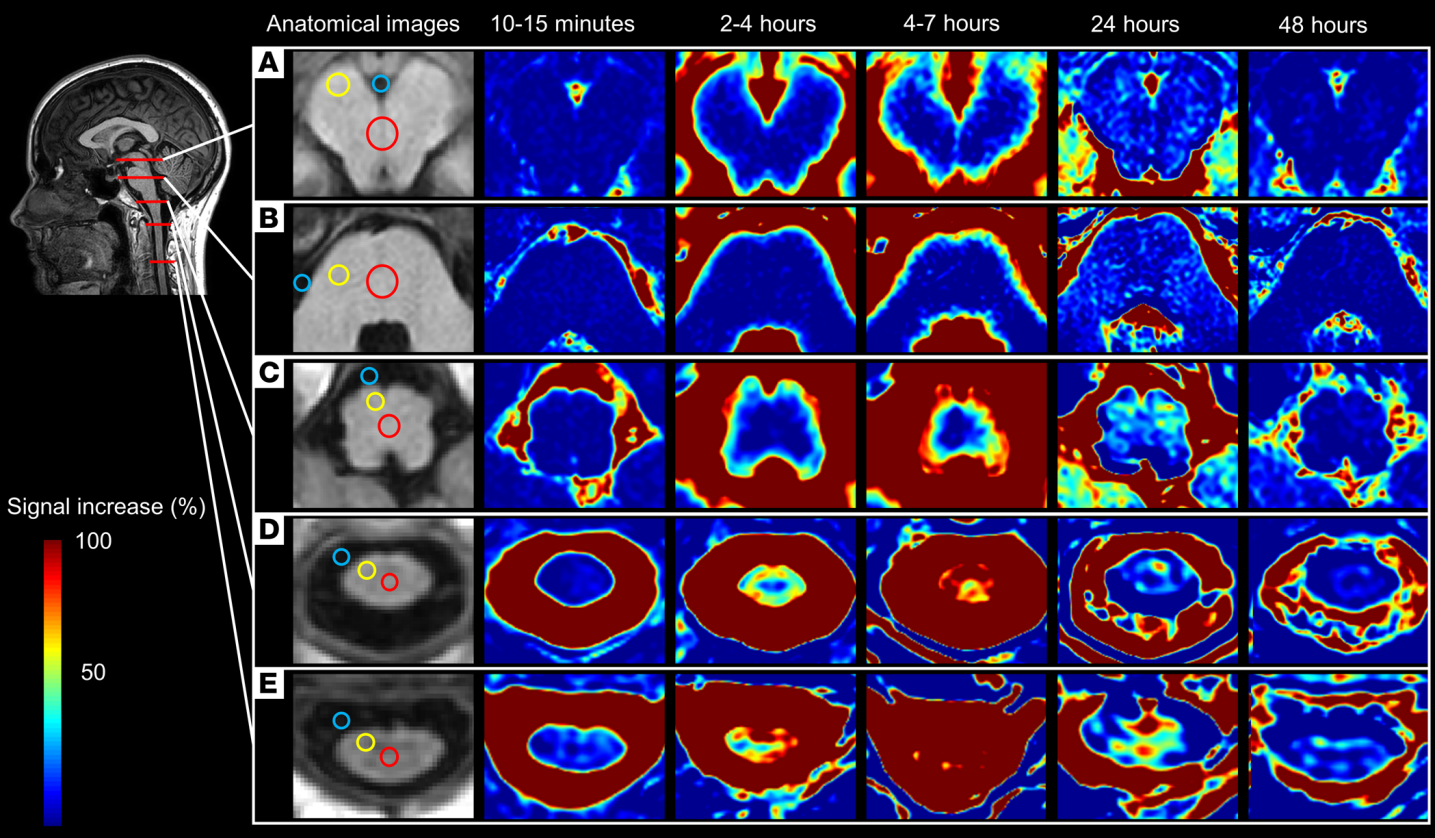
Tracer distribution over time and regions of interest for one reference patient. (A–E) Images of all time points and anatomical regions: mesencephalon (A), pons (B), medulla oblongata (C), C1 level of the spinal cord (D), and C3-level of the spinal cord (E). The 3D T1 images for all time points were first registered using Philips IntelliSpace, version 12.1. The signal in the T1-weighted images before contrast was then subtracted from the normalized signal for each time point. The tracer moves in a centripetal direction with higher and earlier detected levels of tracer in the superficial regions compared with the deep regions. For each time point, 15 regions of interest (ROIs) were placed on axial T1-weighted images in the hospital’s picture and archiving system (Sectra, version 7). The ROIs for each region are marked with circles in the anatomical images: blue represents ROIs in cerebrospinal fluid, yellow represents ROIs in the superficial parts of the parenchyma, and red represents ROIs in the deep parts of the parenchyma.
